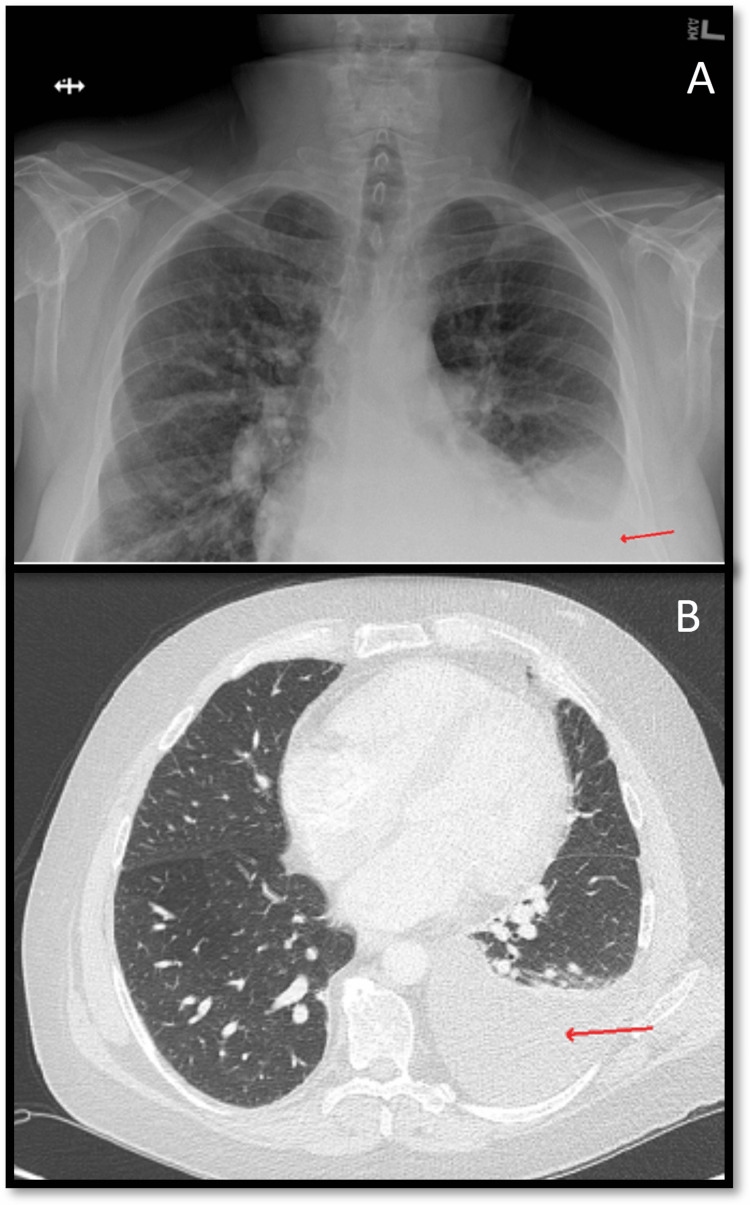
(A) Chest X-ray on admission demonstrates moderate left pleural effusion (arrow). (B) CT chest with IV contrast showing left-sided pleural effusion (arrow) one day after initial thoracentesis without evidence of mass effect or solitary nodules CT: Computed tomography; IV: intravenous
